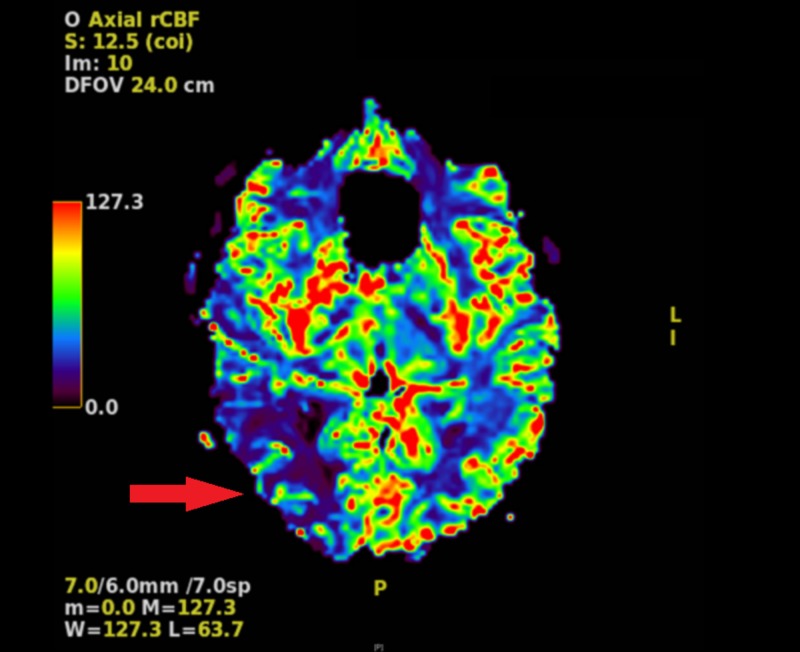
MRI perfusion demonstrating decreased cerebral blood flow in the posterior right hemisphere compared to the left hemisphere (red arrow).
